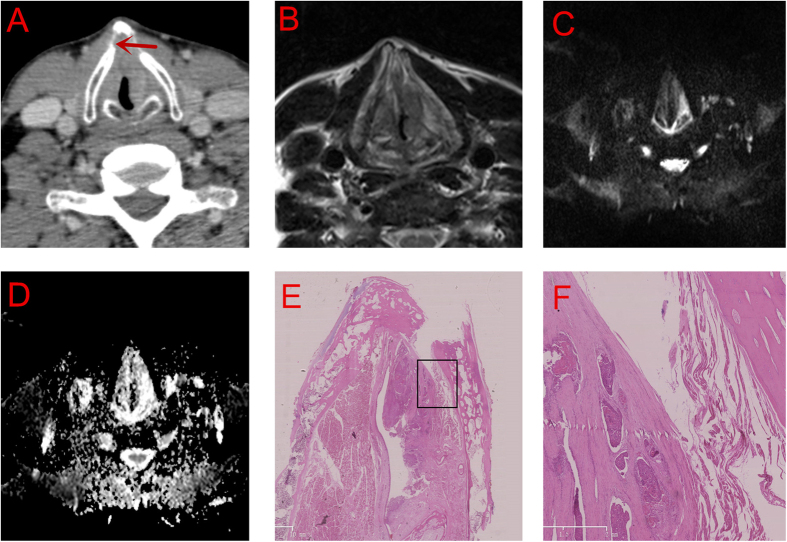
The preliminary study of DWI. (A) CT scan: suspected perichondrium invasion. (B) (T2WI) (C) (DWI) and (D) (ADC) MRI scan: Without involvement of perichondrium. (E,F) (amplified image within the rectangle in E., 200×) Serial section: Without involvement of perichondrium.
